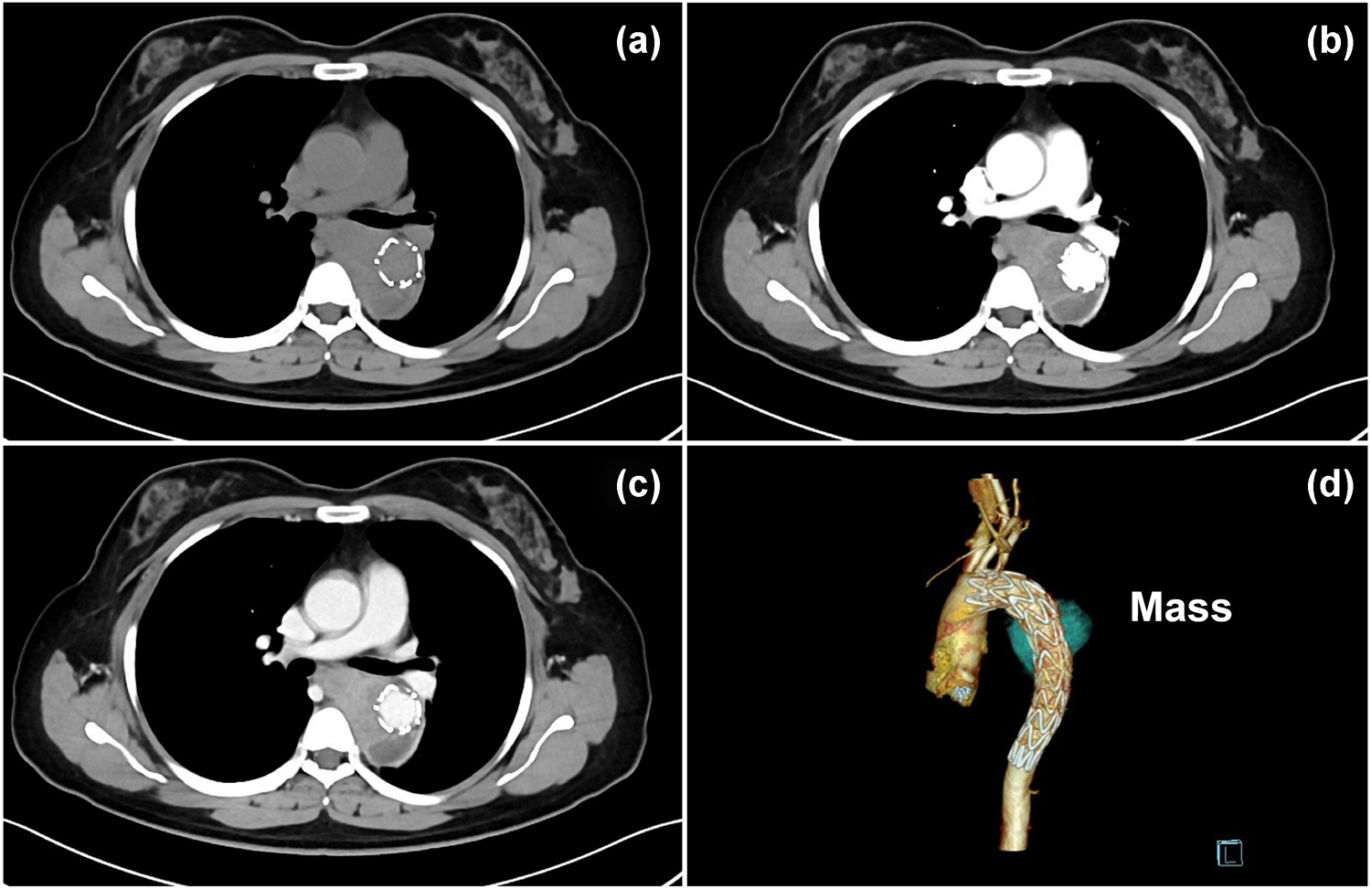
Axial nonenhanced CT image (a) and CTA (b–d) showing enlargement of the false lumen three months after stent implantation, with nonhomogeneous enhancement and mediastinal extensions of the false lumen, which was interpreted by radiologists in our hospital and other medical centers during follow-up as an endoleak and periaortic hematoma.
